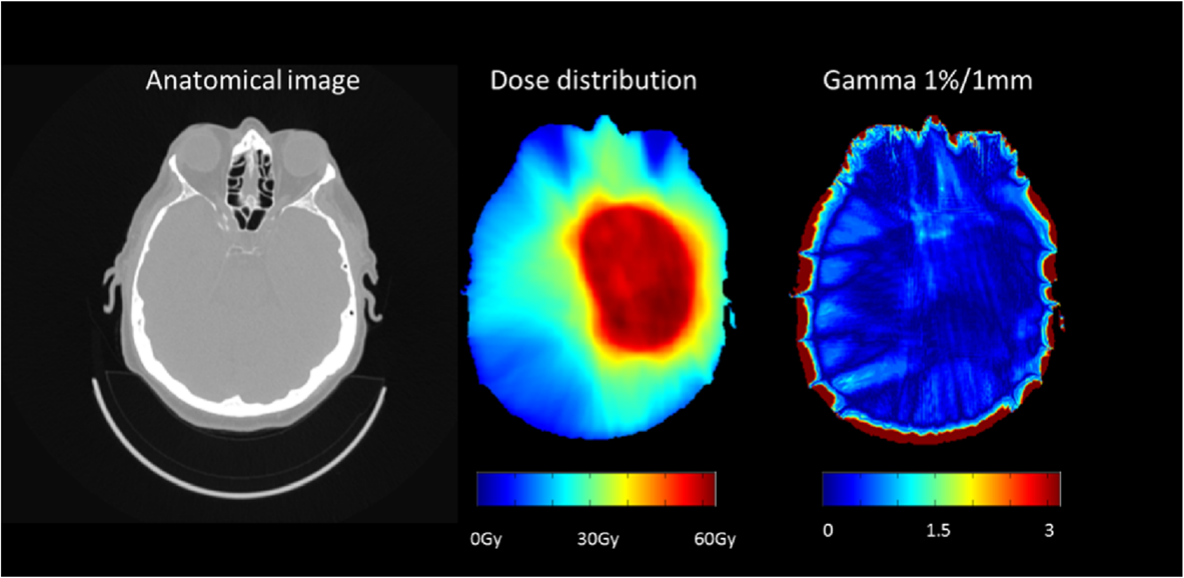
Anatomical CT image, dose distribution and gamma map for a representative slice of the patient with the largest observed dosimetric errors. The high dose region extends into the ethmoidal and sphenoidal sinuses.
